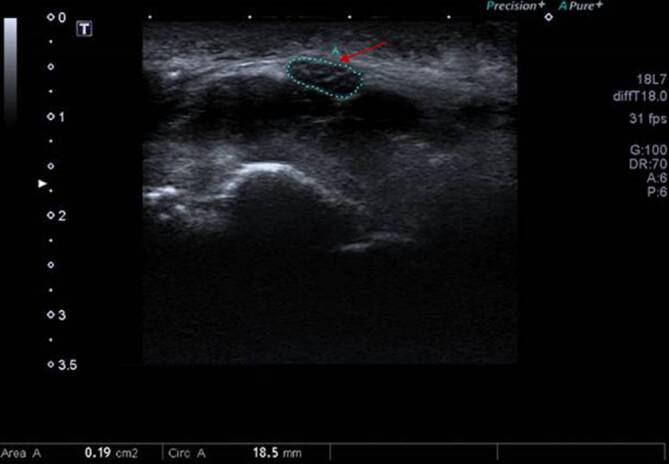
Nervensonographie 02/2024: Es zeigt sich ein klassischer Befund eines Karpaltunnelsyndroms mit Schwellung des N. medianus (siehe *roter Pfeil* und *grün gepunktetes Oval*) vor dem Eintritt in den Karpaltunnel
